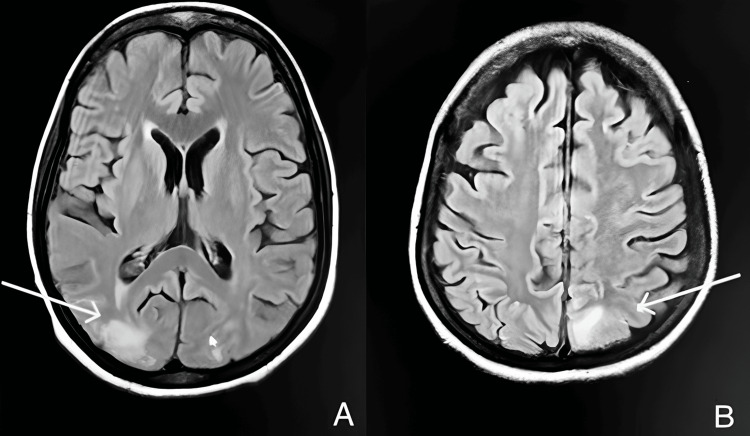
MRI findings consistent with atypical posterior reversible encephalopathy syndrome. (A) Axial T2-weighted and (B) fluid-attenuated inversion recovery MRI showing hyperintensities involving the cortical and subcortical white matter of the bilateral parieto-occipital regions. These findings are consistent with atypical posterior reversible encephalopathy syndrome.
